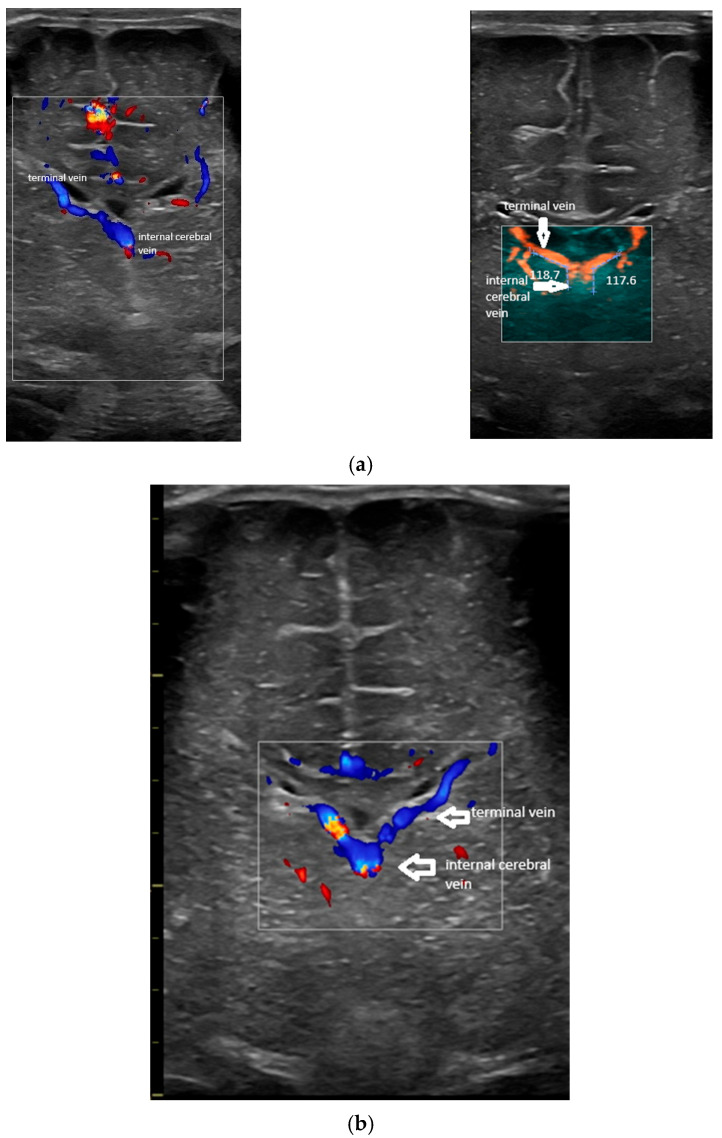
(a) Coronal section at the foramen of Monro (power Doppler). Normal pattern; confluence of the terminal vein and internal cerebral vein at the level of the foramen of Monro; patient with multicystic images in the caudo-thalamic groove, bilateral; GM/IVH grade 1 in evolution. (b) Colour Doppler (same section). The course of the terminal vein can be followed until the confluence with the internal cerebral vein.
